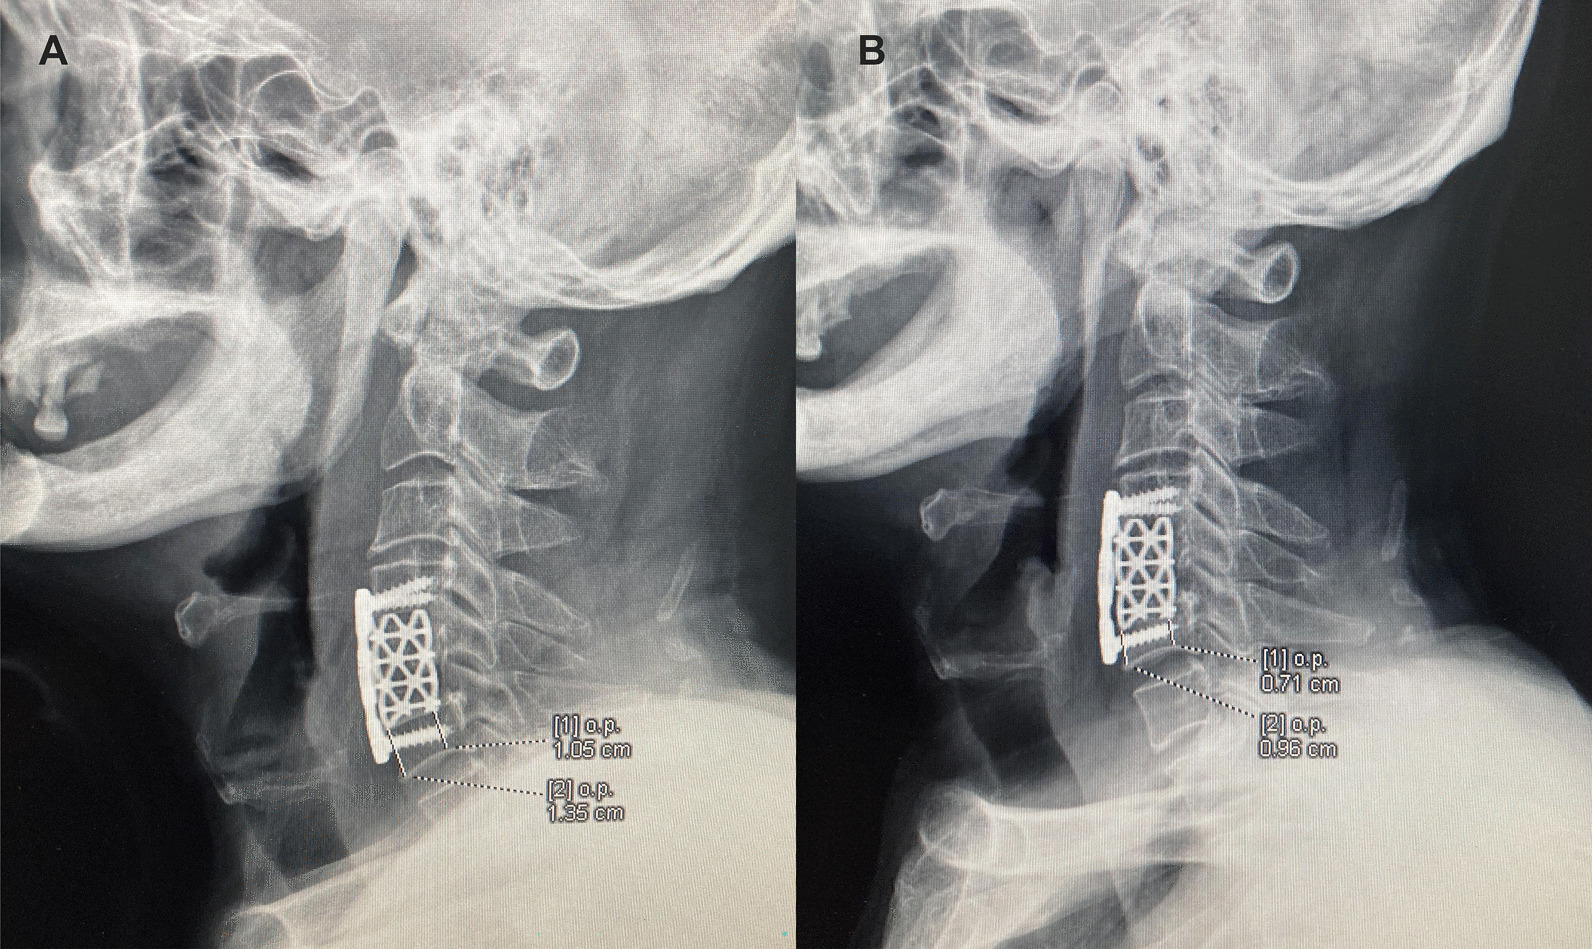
Compare immediately (A) and within 3 months (B) after surgery at lateral X-ray to calculate the TMC subsidence
